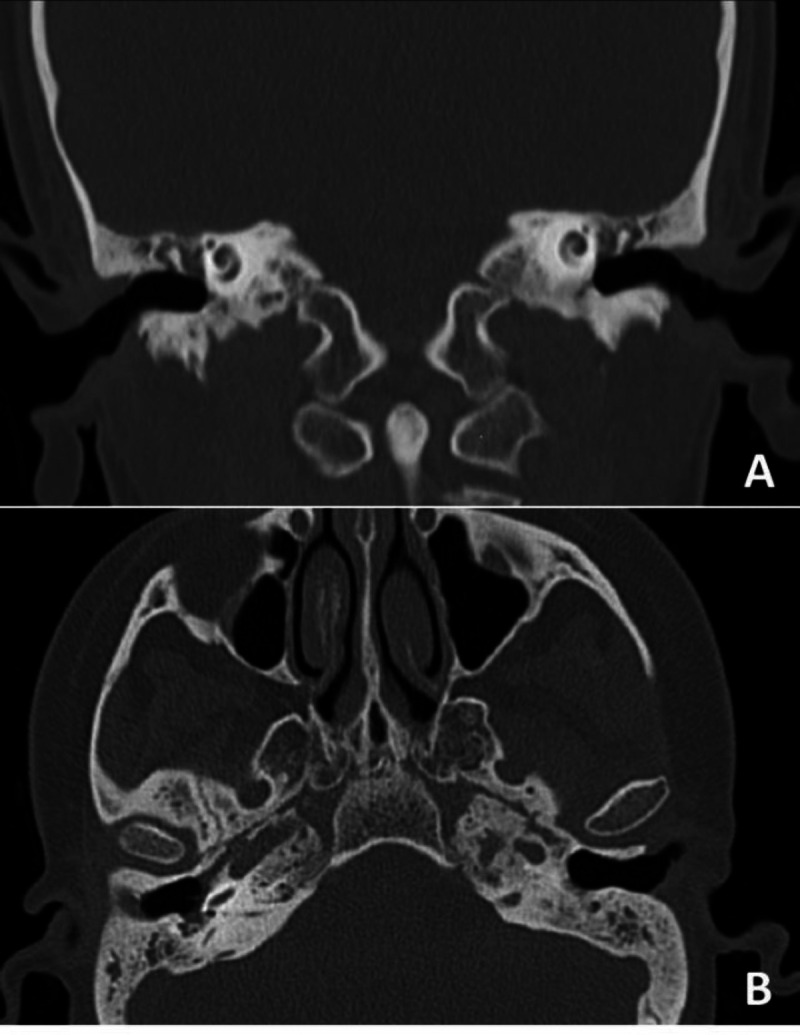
Pre-operative temporal CT scan. A) coronal view, B) axial view
